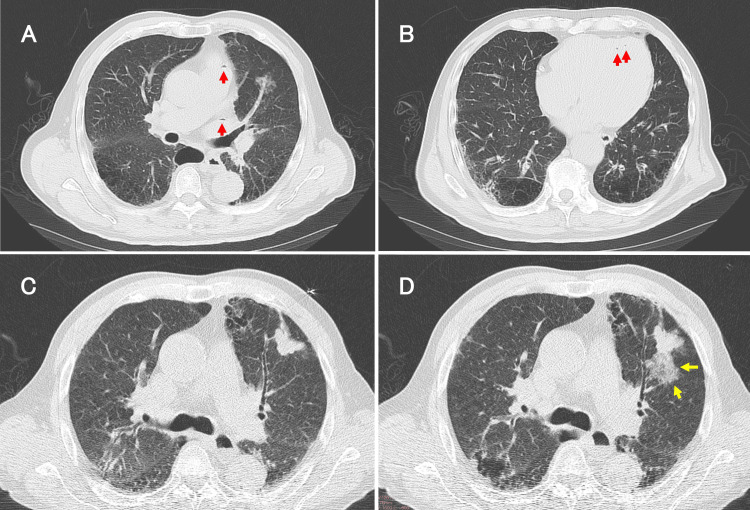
Chest CT images during CT-guided lung needle biopsy in case 2. Axial chest computed tomography images post lung needle biopsy, showing air within the pulmonary artery and vein (A) and left ventricle (B). At the beginning of the examination, there was no bleeding around the nodule (C); however, bleeding occurred during the examination (D).
